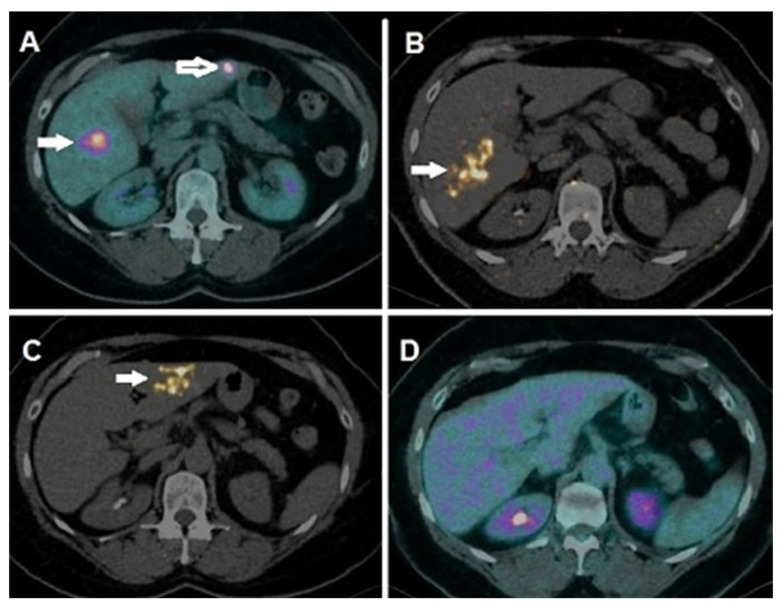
(A) Pre-SIRT 18 F-FDG PET/CT demonstrated intense focal tracer uptake in two hepatic lesions located in the 6th (white arrow) and 3rd (white-contoured arrow) segment, respectively. (B) PET/CT acquired on 90 Y-positronic photopeak after the 1st SIRT lobar session showed optimal targeting of the right hepatic lobe and metastasis (white arrow). (C) PET/CT acquired on 90 Y-positronic photopeak after the 2nd SIRT lobar procedure depicted adequate targeting of the left hepatic lobe’s metastasis (white arrow). (D) Four months after SIRT, 18 F-FDG PET/CT showed a complete metabolic response.
